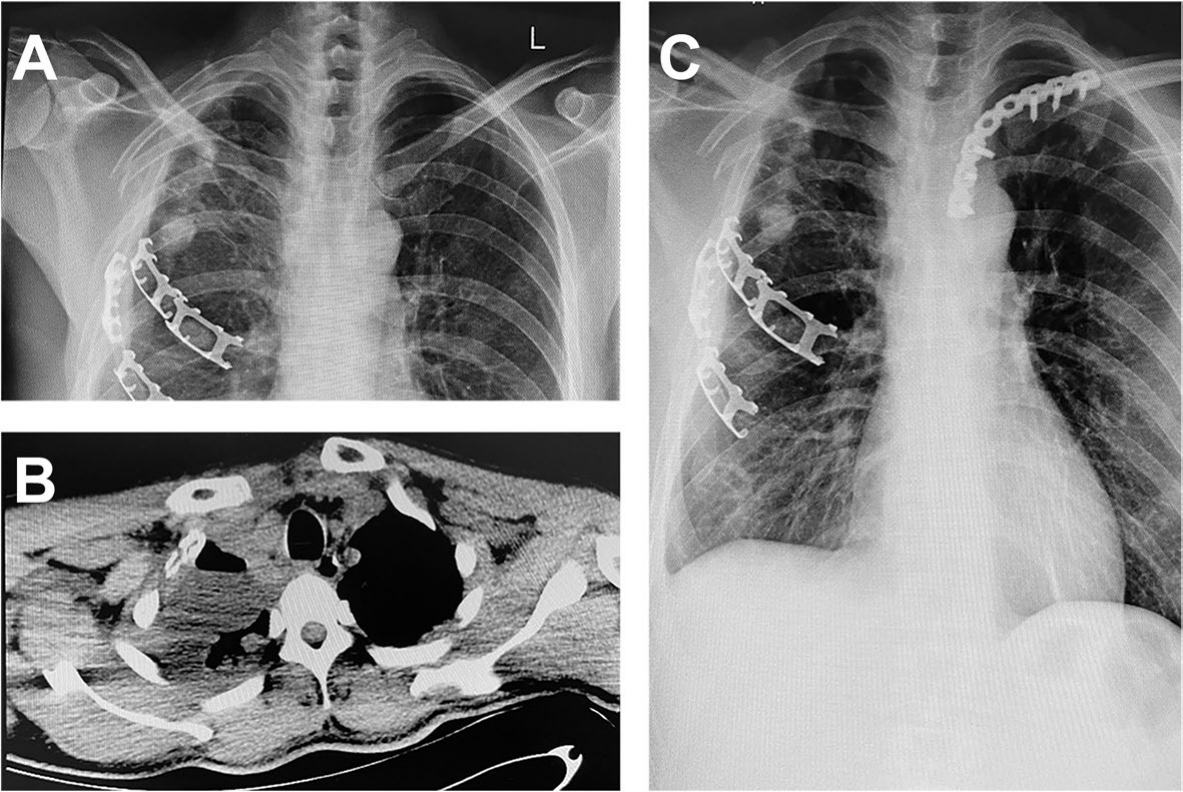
Left anterior sternoclavicular joint dislocation treated with a locking plate (LP). A Preoperative radiograph of the patient. B Preoperative computed tomography (CT) scan shows a dislocation of the left anterior sternoclavicular joint. C Postoperative radiograph of the patient shows good reduction of the left sternoclavicular joint
